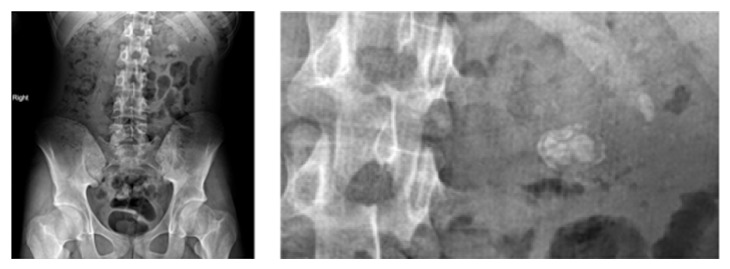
Preoperative KUB radiography of patient.
